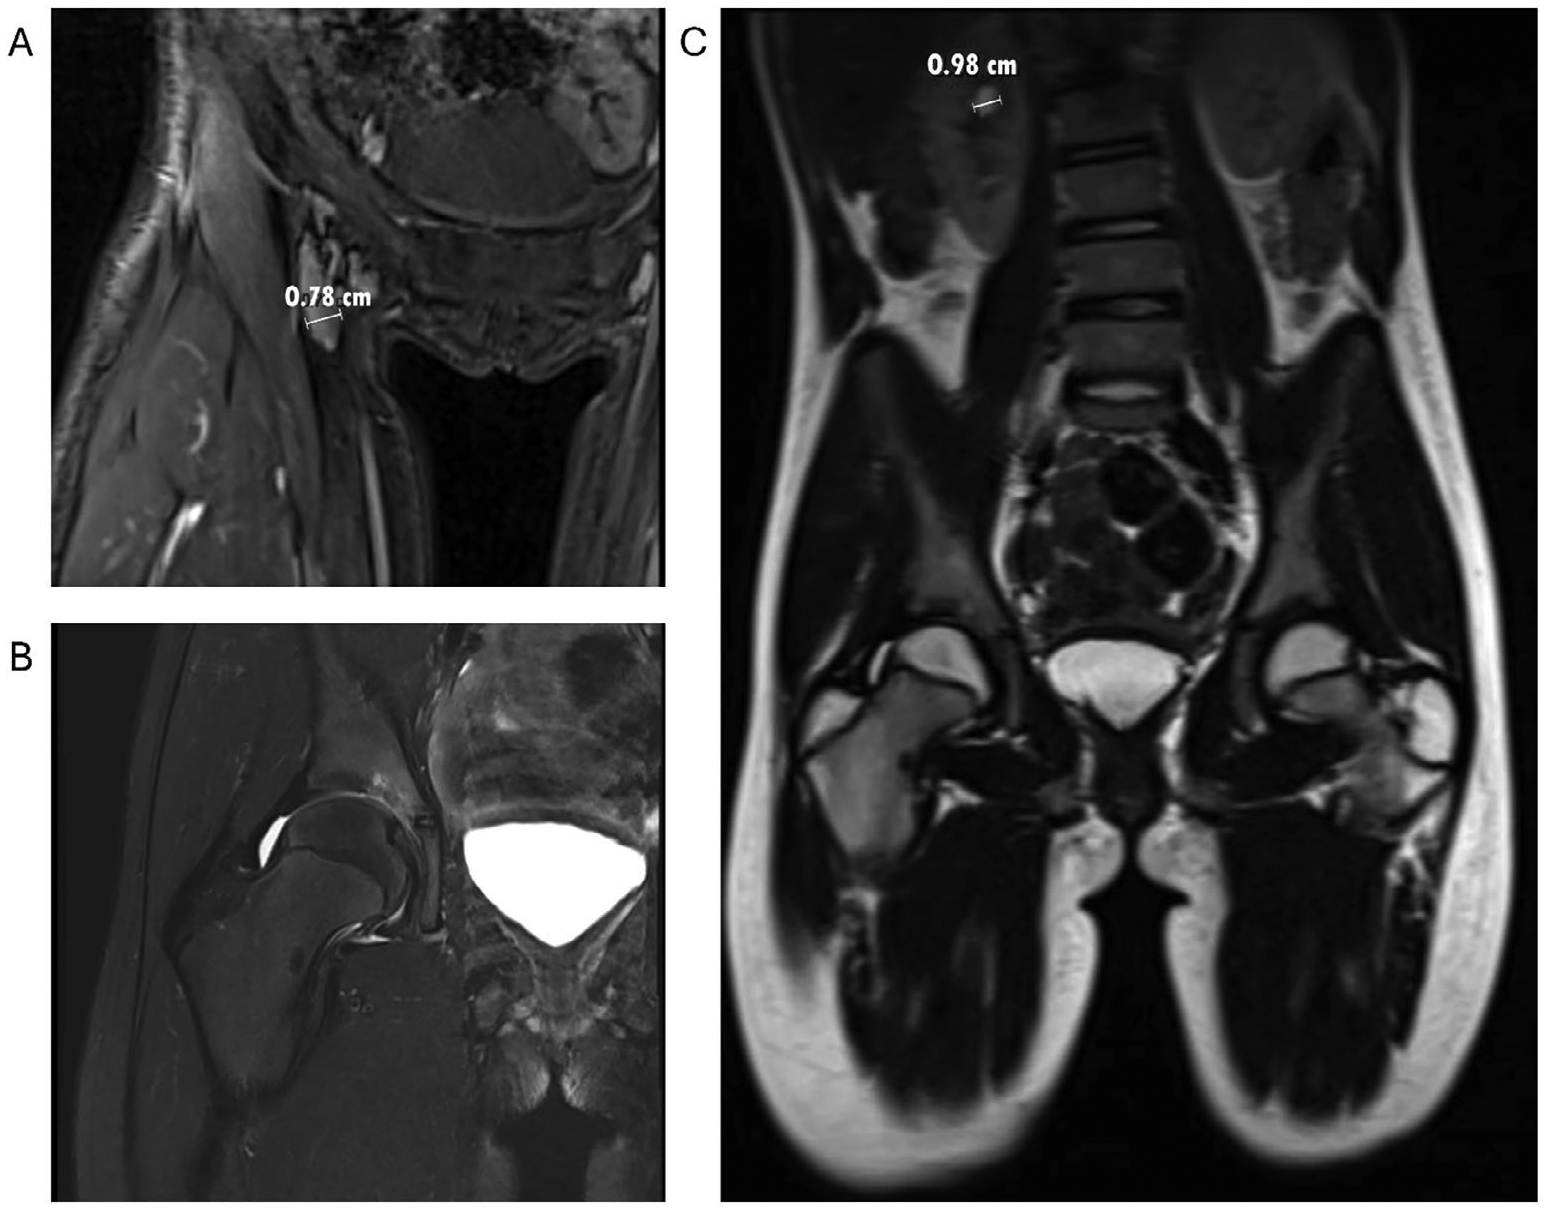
Multiplanar multisequence MRI of the right hip with and without contrast. (A, B) Small to moderate right hip joint effusion with synovitis/debris and minimal synovial enhancement. (C) Scout imaging with partially visualized right upper pole parapelvic cyst measuring approximately 1 cm.
